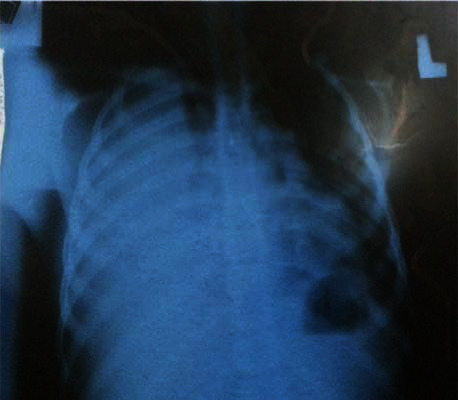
Chest roentgenography of the patient showing massive right pleural effusion.
